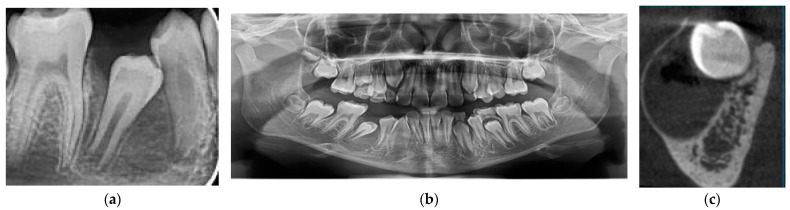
Case 2’s initial preoperative images: (a) retro alveolar radiography; (b) panoramic view showing the discrete encircling radiolucency; (c) cortical expansion.
